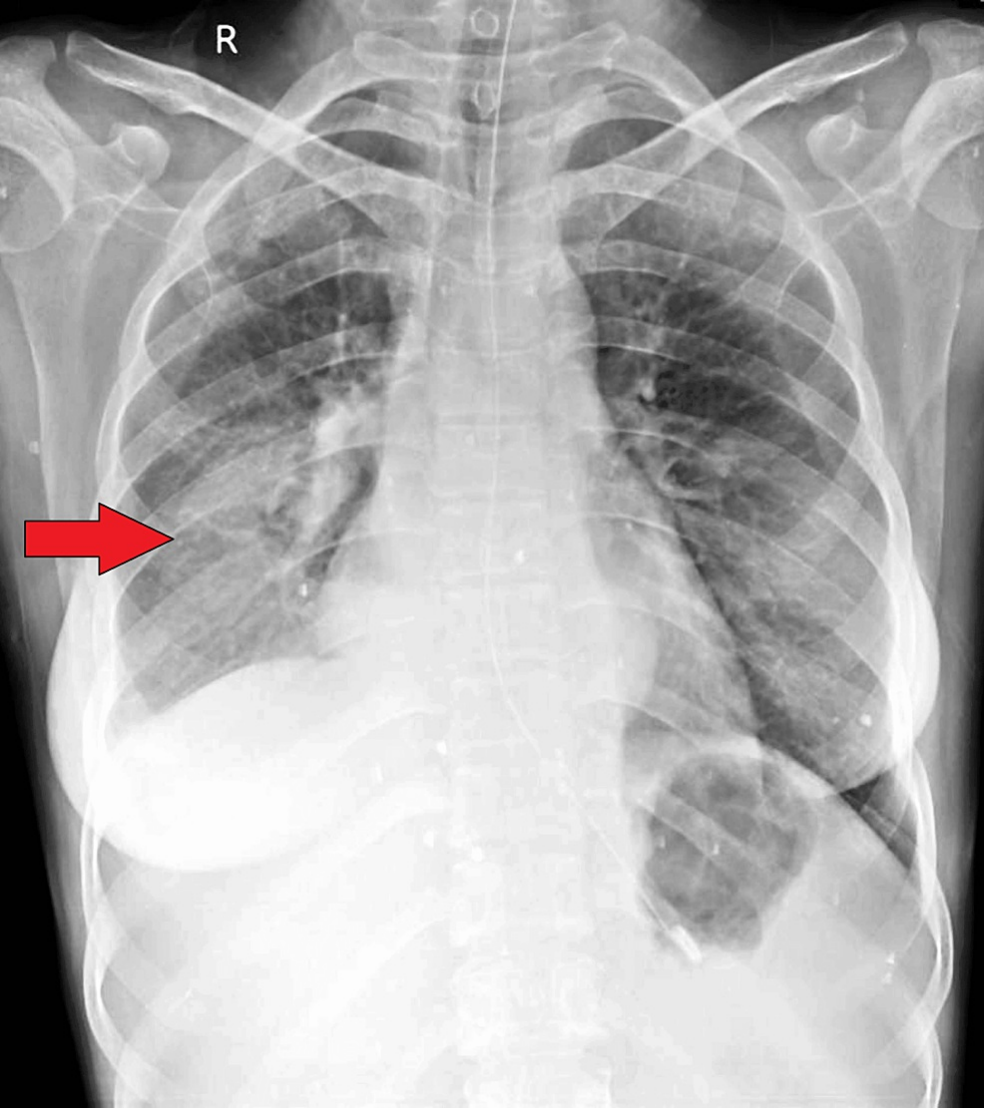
A chest X-ray done on admission showing homogenous opacity in the right lower lobe
